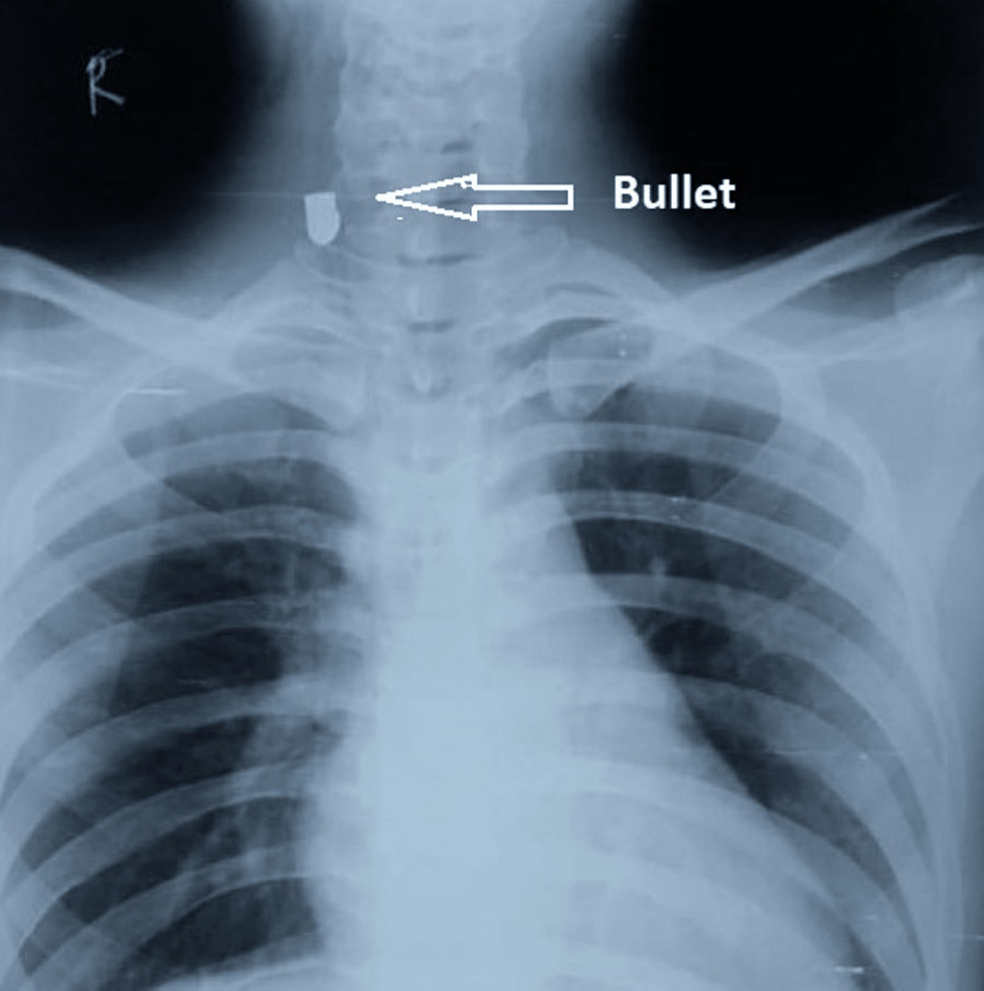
Bullet at C6-C7 level in antero-posterior view of x-ray neck and chest.
